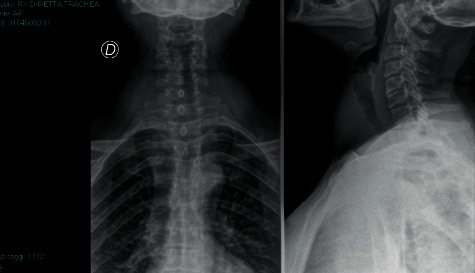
Direct trachea X-ray (Case 2).
